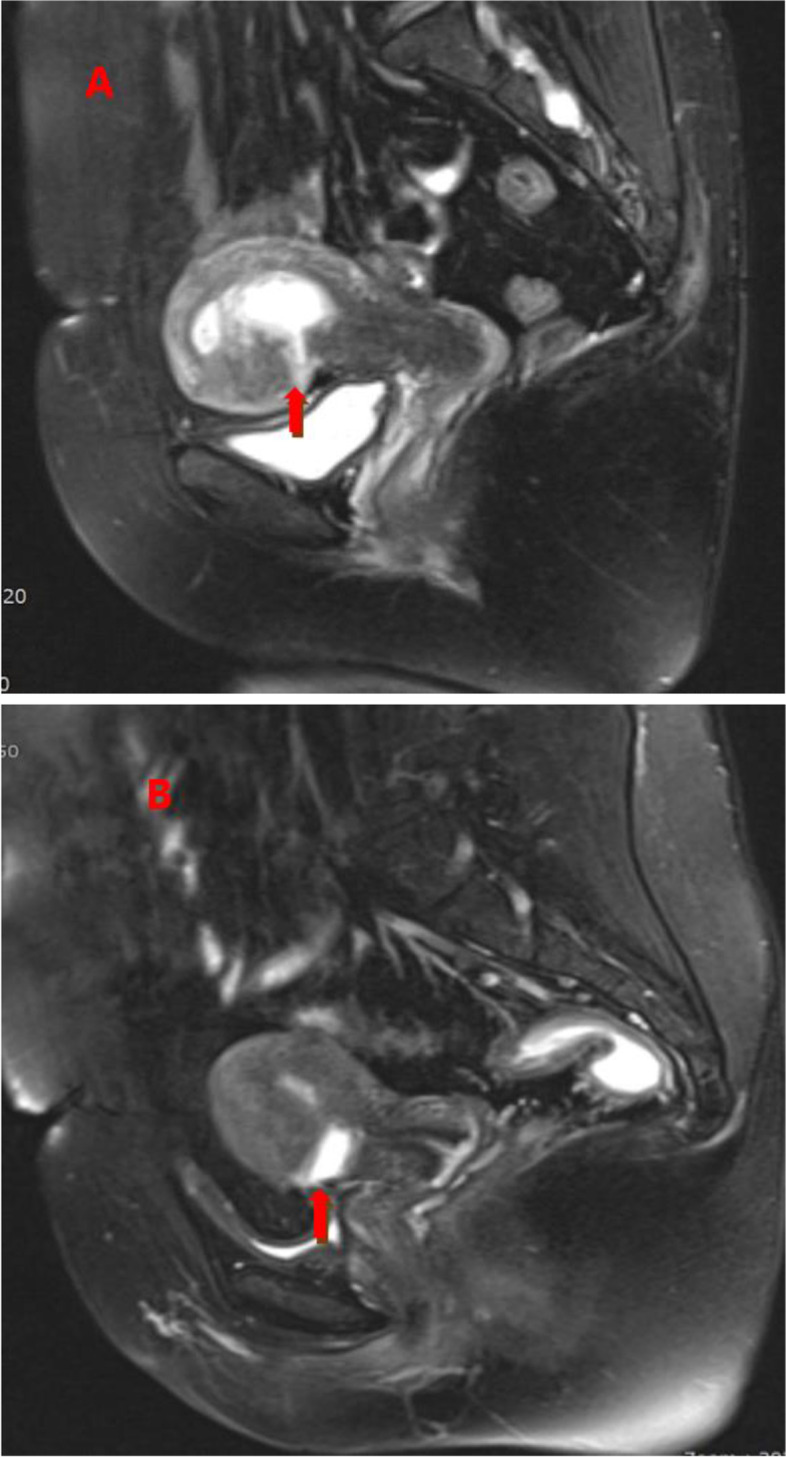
MR images of the patients with CSP. A MRI image shows the amniotic sac on the scar with progression of the pregnancy implanted in the isthmus uteri and in the uterine cavity (arrow). B MRI image shows the amniotic sac deeply implanted into previous cesarean scar defect with infiltrating growth into the uterine myometrium (arrow)
